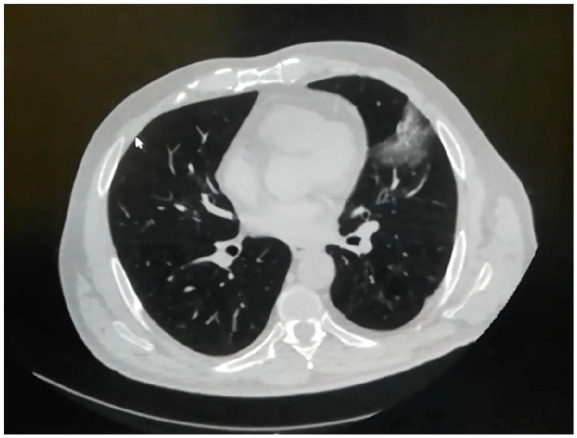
Thorax CT scan: left apical interstitial pneumonia.
